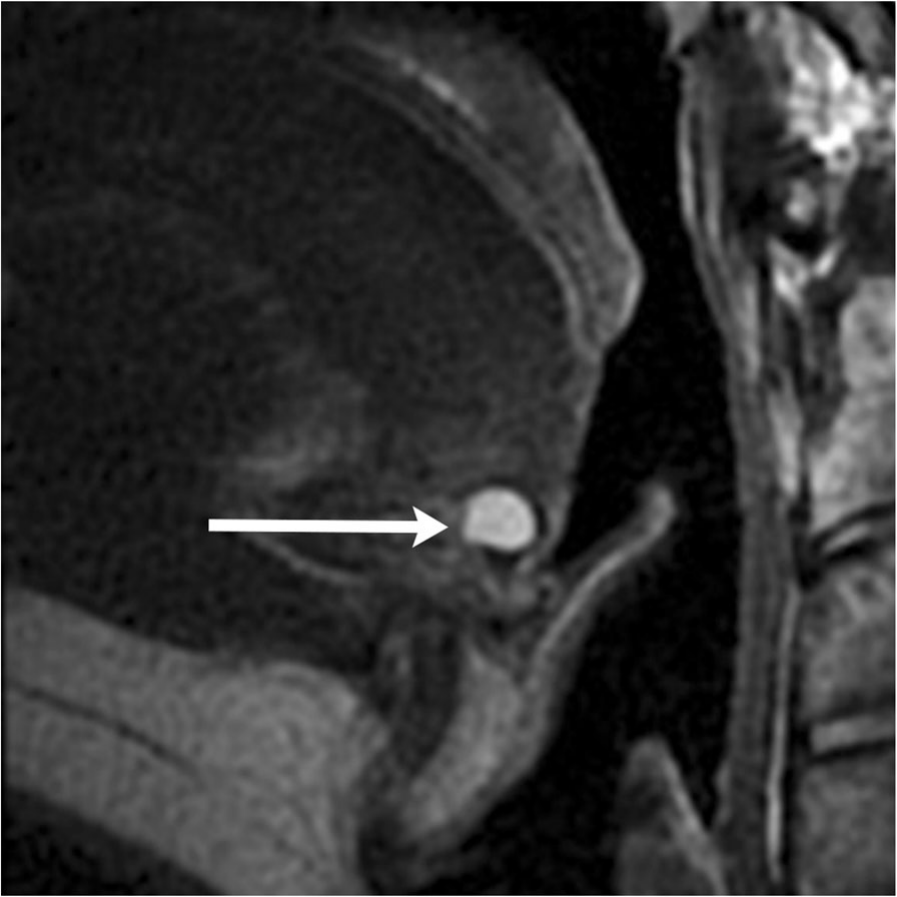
Lingual thyroglossal duct cyst. Sagittal T2-weighted MR image demonstrates a fluid signal lesion at the foramen cecum (arrow)
